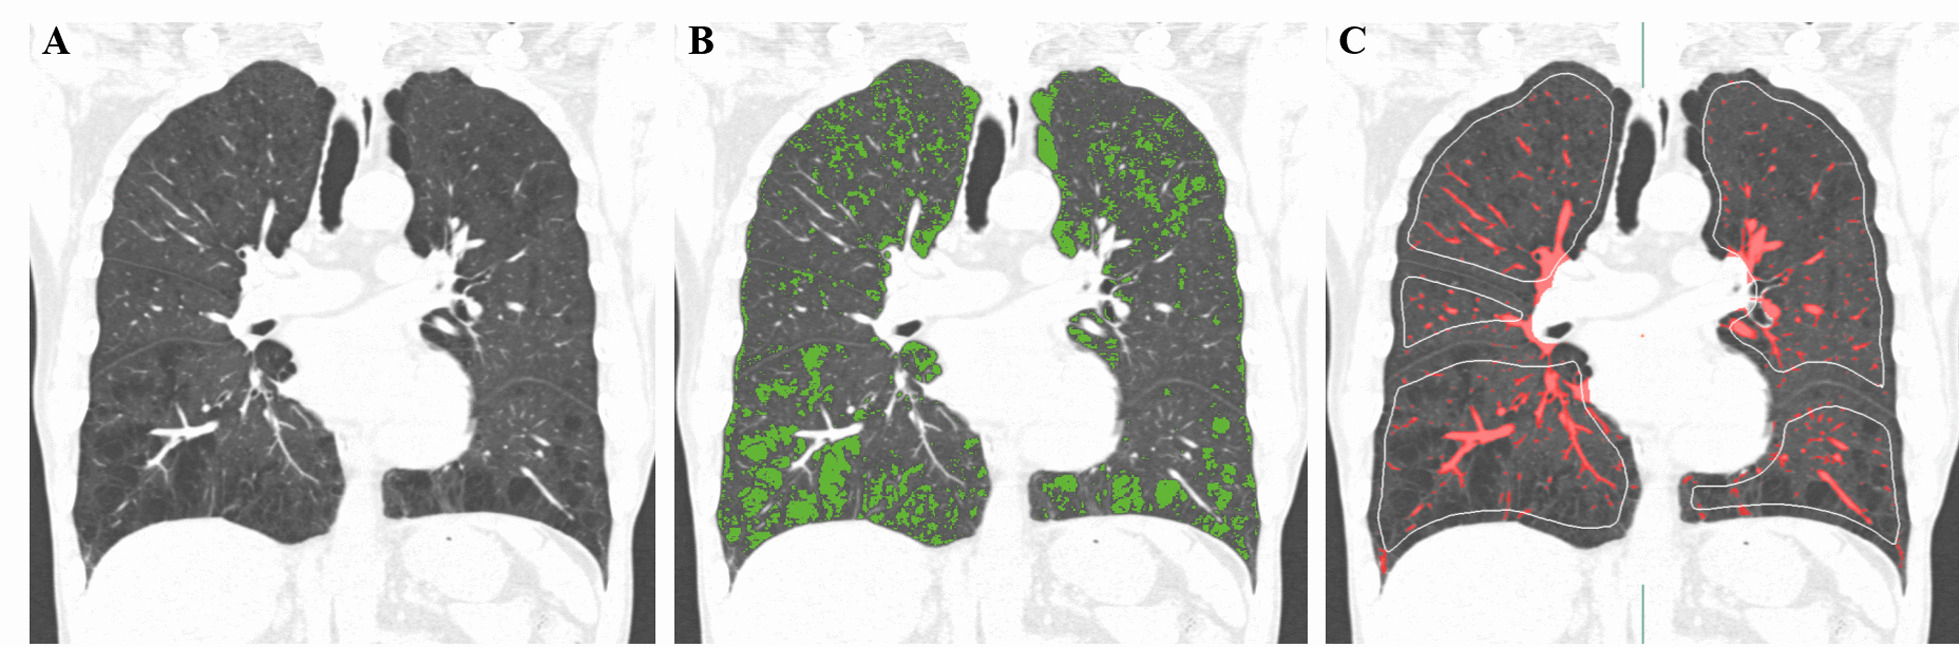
CT quantification of emphysema and pulmonary vessels. A CT coronal reconstructed image of a 54-year-old male with COPD GOLD grade 1 reveals centrilobular and paraseptal emphysema. B The emphysema was measured using a threshold of -950HU (shown in green; LAA-950, 12.3%). C Pulmonary vessels are automatically extracted and segmented (shown in red; Ntotal/LSA, 1.05; N5mm/LSA, 0.97), and the green contours show the lung surface area at 6 mm from the pleural surface. *CT* computed tomography, *LAA* low attenuation area
